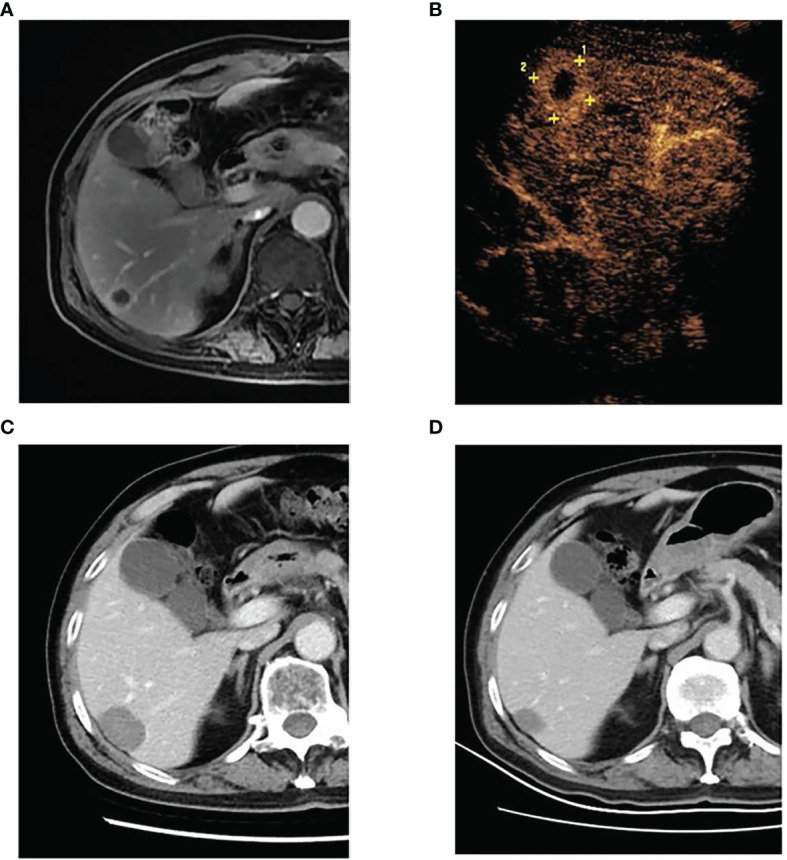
A 68-year-old man was diagnosed with left lung adenocarcinoma and received radiotherapy and chemotherapy for the lung lesion. After 27 months, metachronous liver metastasis was detected by magnetic resonance imaging. The patient underwent percutaneous radiofrequency ablation for liver metastasis. Until the last follow-up, the patient was alive, surviving 30 months without recurrence. (A) The liver metastasis in segment VI, as detected by magnetic resonance imaging. (B) Contrast-enhanced ultrasound showing a 2.1-cm liver tumor in segment VI. (C) Contrast-enhanced computed tomography at 1 month after radiofrequency ablation demonstrating the successful ablation of the tumor. (D) Contrast-enhanced computed tomography at 30 months after radiofrequency ablation showing that the ablation zone shrunk without local recurrence or new liver metastases.
